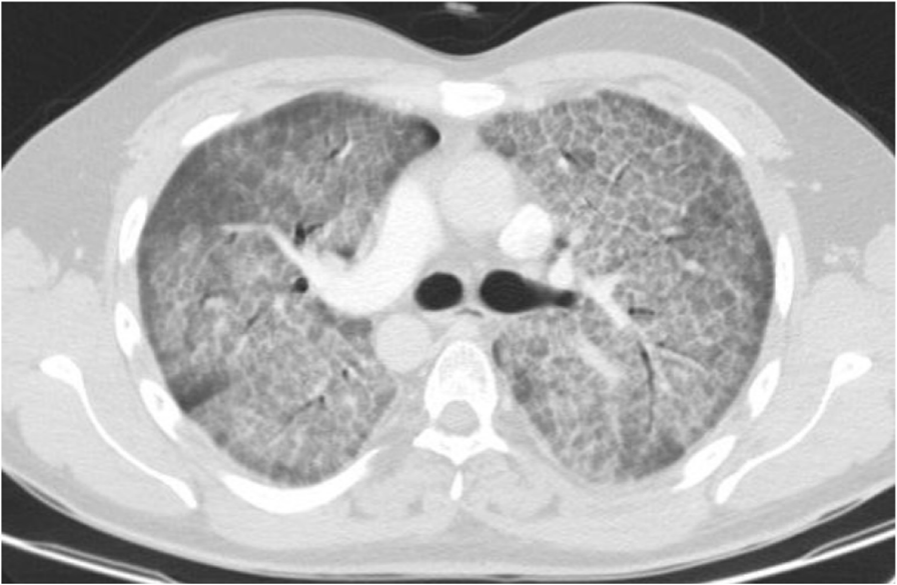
Pulmonary alveolar proteinosis: thin section CT shows bilateral GGO and reticulation (crazy-paving appearance)
